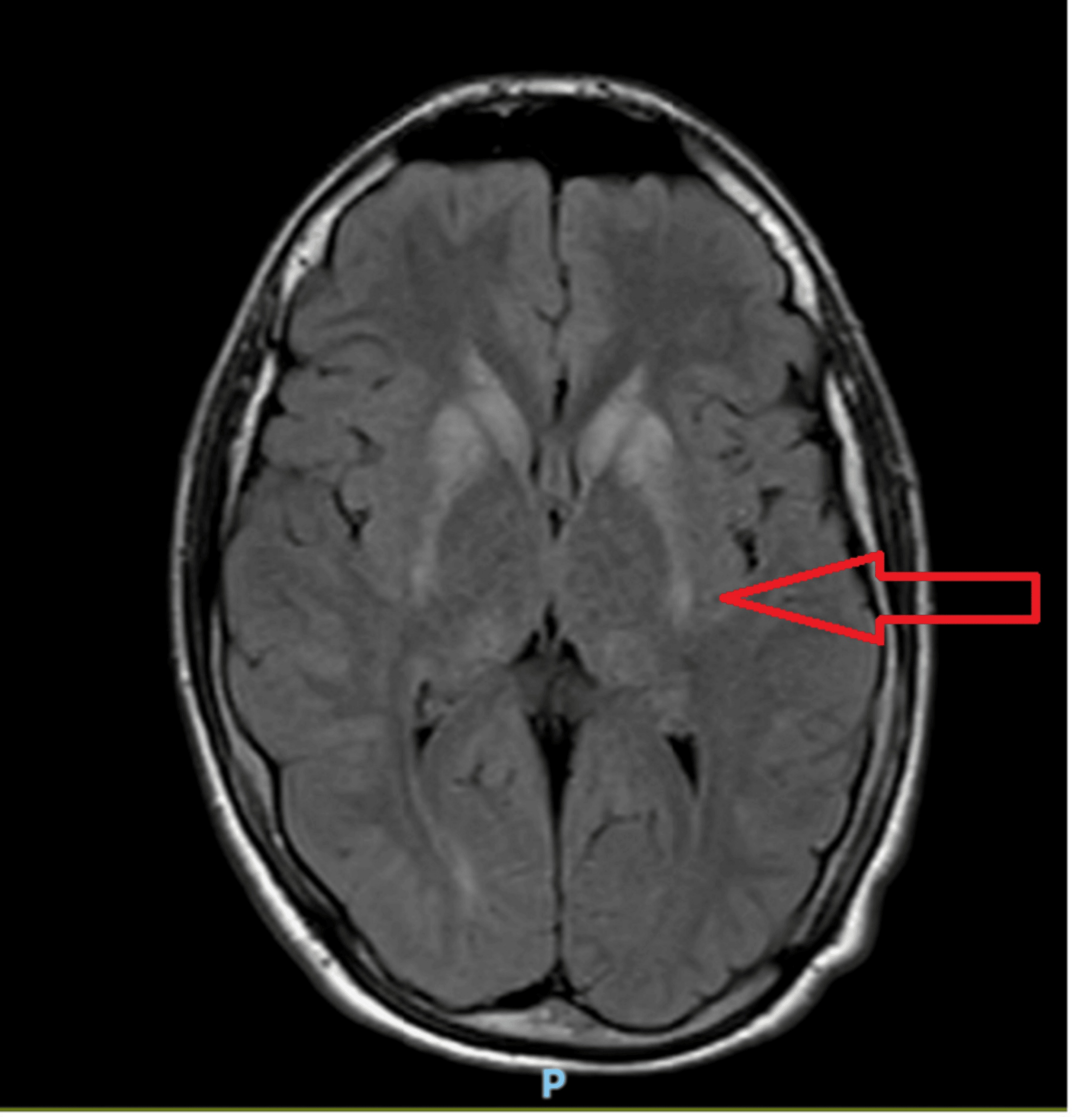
Axial FLAIR MRI of the brain demonstrating symmetrical signal changes in the bilateral basal ganglia and dentate nuclei, consistent with possible calcification (red arrow), one of the characteristic features of PHP. PHP: pseudohypoparathyroidism; FLAIR: fluid-attenuated inversion recovery
